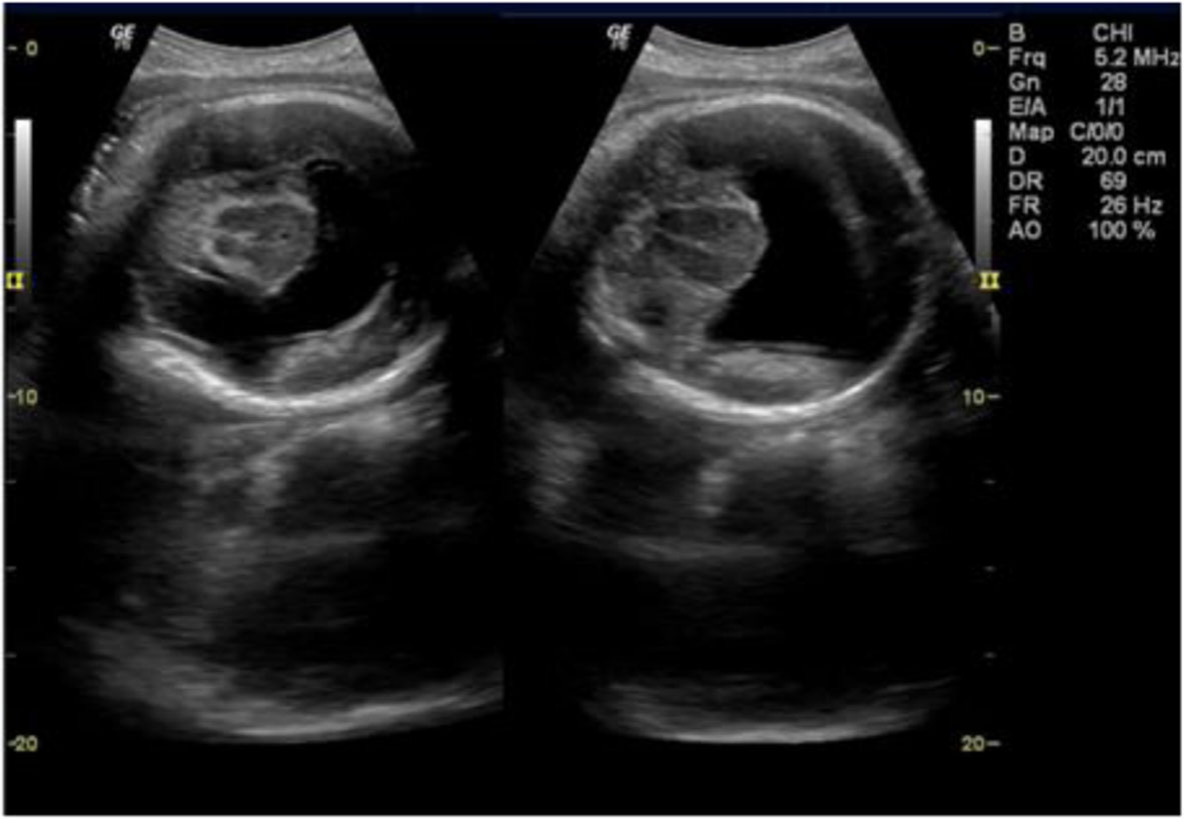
The above images were from a mother in the age range 30–34 years at 38 weeks 5 days gestation who presented with a history of decreased fetal movements. On ultrasound examination, the head showed fused thalami, a monoventricle/holosphere with absent interhemispheric fissure. A diagnosis of alobar holoprosencephaly was made. The other systems were normal. Mortality occurred on the second day post-delivery in the neonatal intensive care unit (NICU)
